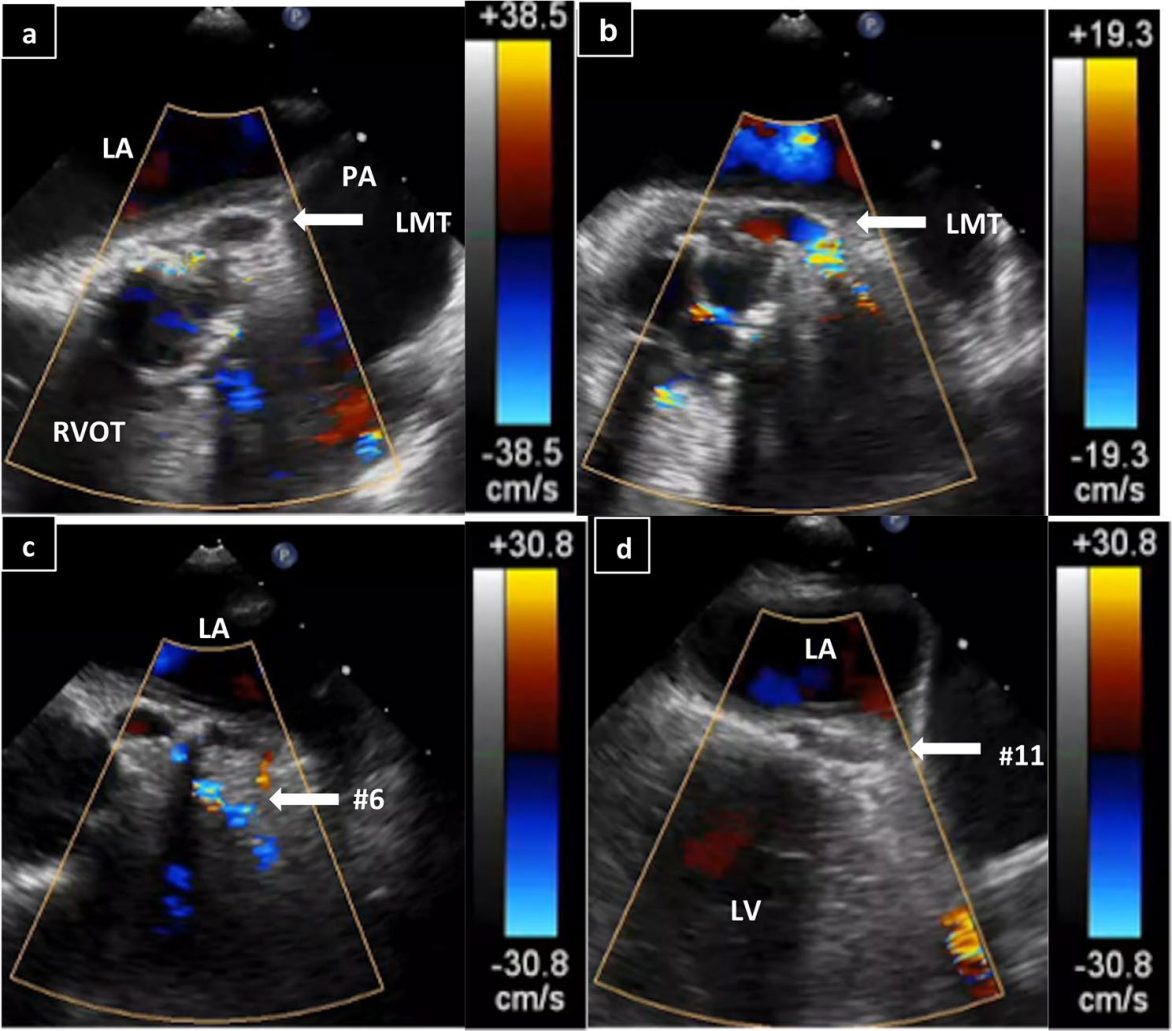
a, b, c, d. Transesophageal echocardiogram findings after asynergy. The left main trunk blood flow became undetectable in the range of 38.5 cm/s; 30-degree scanning angle. (a), but was picked up by reducing the blood flow range to 19.3 cm/s; 30-degree scanning angle. (b). Proximal left anterior descending artery (#6) flow was narrowly detectable; 30-degree scanning angle. (c), and no distal circumflex artery (#11) flow was observed; 120-degree scanning angle. (d)
